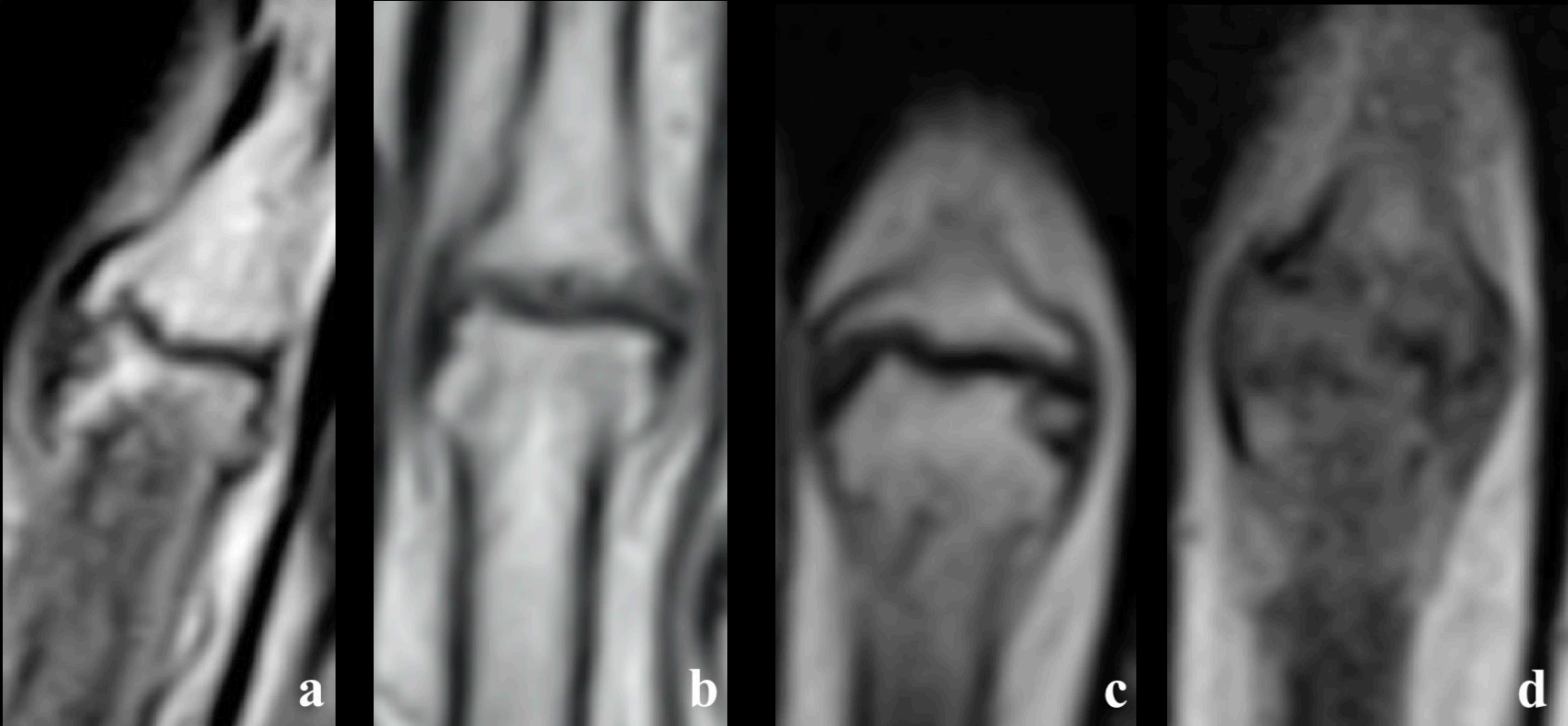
MRI joint structural features. a.: osteophytic feature (O) b.: erosive feature (E) c.: erosive and osteophytic lesion feature (E/O) d.: complete articular destruction (D).
